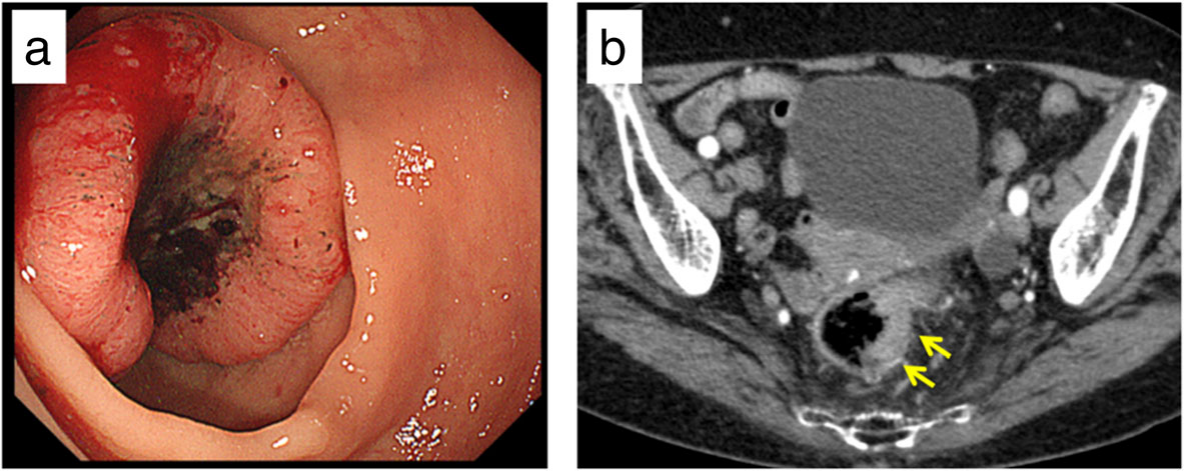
Preoperative findings. a Preoperative colonoscopy shows a type 2 tumor localized in the upper rectum. b An abdominal contrast CT scan shows wall thickening of the rectum (yellow arrows)
